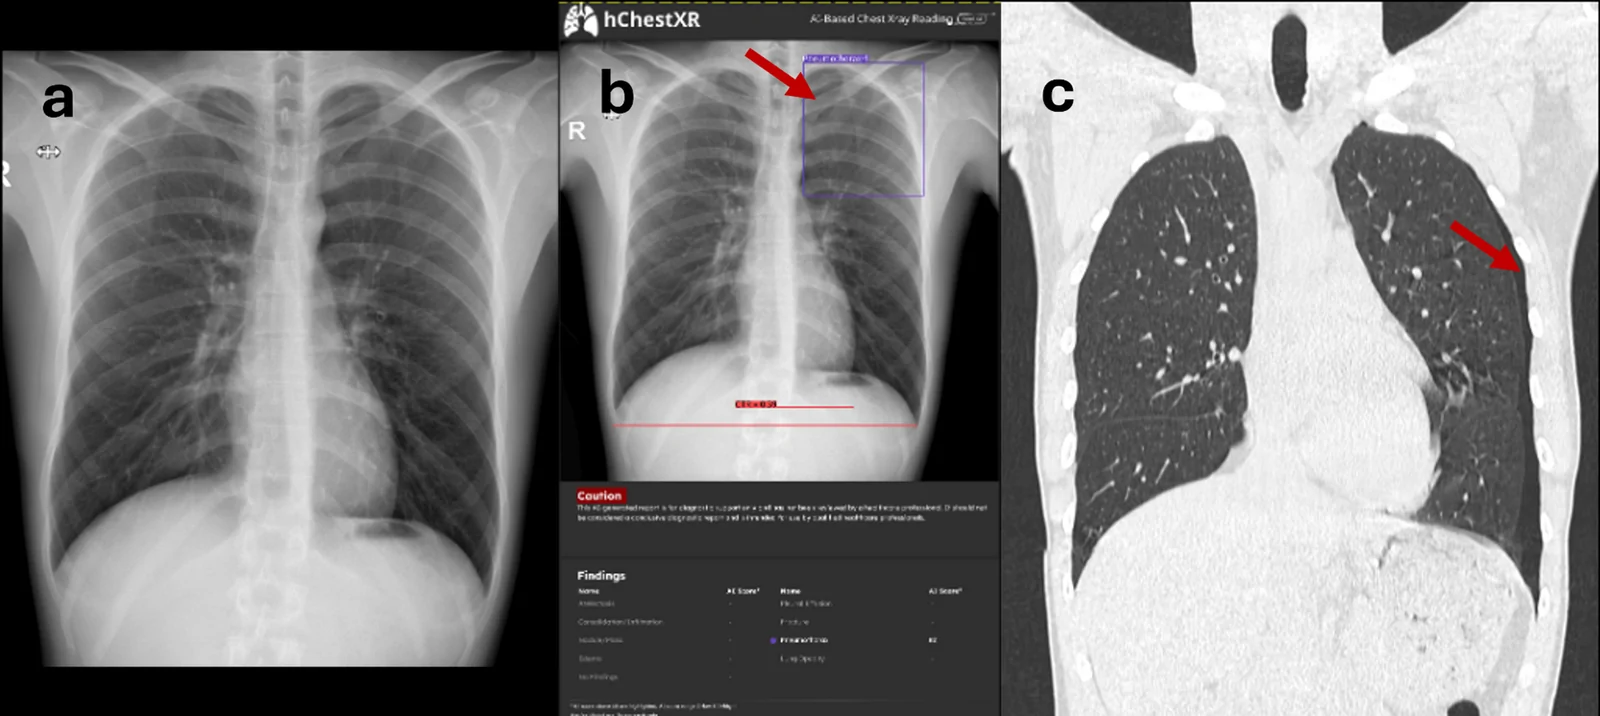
A 30-year-old male patient presented to the emergency department with chest pain in Center D. (a) Posteroanterior chest radiograph of the patient. (b) The AI system identified the pneumothorax in the left upper zone (arrow). (c) The corresponding coronal CT image demonstrates the pneumothorax in the left hemithorax (arrow). This case was classified as true positive.
